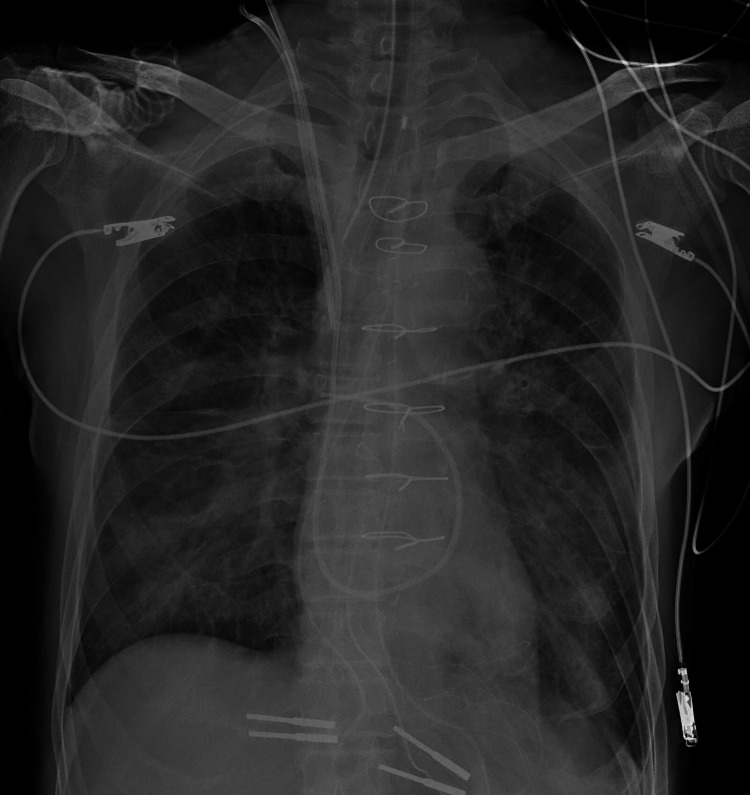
Chest X-ray at the onset of severe hypoxemia in the intensive care unit
